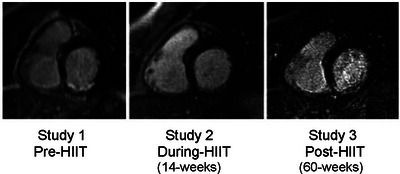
Three still images, each taken from functional cardiac MRI (i.e. cine images). Data collected were Study 1, November 2008; Study 2, May 2011; and Study 3, March 2012.
